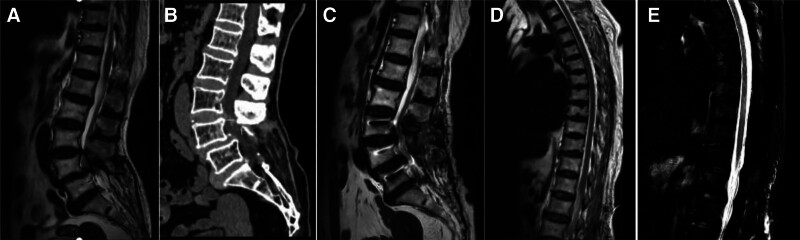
This patient, female, 69 years old. (A) The patient had preoperative MRI of lumbar vertebrae, with anterior spondylolisthesis (I) of lumbar vertebrae 4, posterior protrusion of lumbar intervertebral discs 3 to 4 and 4 to 5, thickening of ligamentum flavum at the same level and stenosis of spinal canal. (B) Before operation, the lumbar spine was scanned by CT, and the L4 vertebral body moved forward and slipped. The lack of bone in L5 partial laminae is the evidence of previous lumbar surgery. (C) Four hours after the lumbar operation, the patient suffered from sensory and motor disorders of both lower limbs and underwent emergency MRI. A slight hematoma was seen around the dura mater in the 3 to 4 plane of the waist. (D) MRI of thoracic spine showed that the shape and signal of thoracic spinal cord were normal, no obvious thickening or thinning and no abnormal changes were found 2 days after lumbar surgery. (E) On the 17th day after the lumbar operation, MRI of thoracic vertebra showed a small round T2W1 signal with slightly higher signal shadow and T2- fat suppression imaging in the horizontal spinal cord of thoracic vertebra 10, and myelitis was considered. CT = computed tomography.
